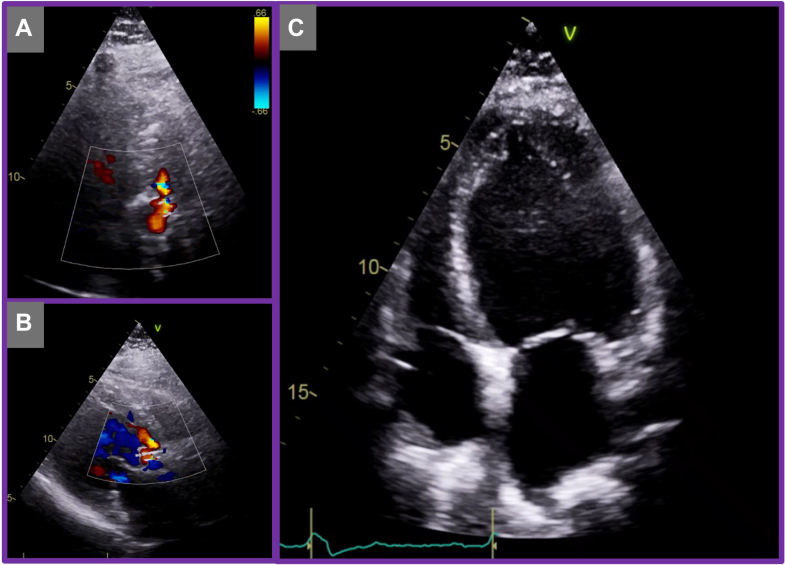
Transthoracic Echocardiography at 10 Months Post-Transcatheter Aortic Valve Replacement (A and B) Demonstrating severe aortic regurgitation. (C) Demonstrating dilated severe left ventricular systolic dysfunction.
